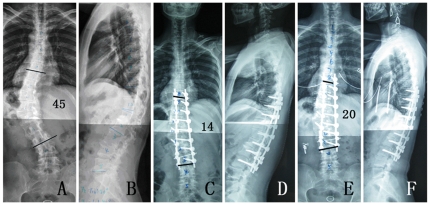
41-year-old female with a BMI of 27.7 diagnosed with thoracolumbar idiopathic scoliosis. (A) anterior-posterior standing and (B) standing lateral full-length spinal preoperative X-rays showing coronal imbalance and sagittal thoracolumbar kyphosis; (C) anterior-posterior standing and (D) standing lateral full-length spinal X-rays: the first post-surgical erect X-rays showing the correction and fusion; (E) anterior-posterior standing and (F) standing lateral full-length spinal X-rays: the two-year follow-up X-rays showed a good balance.
